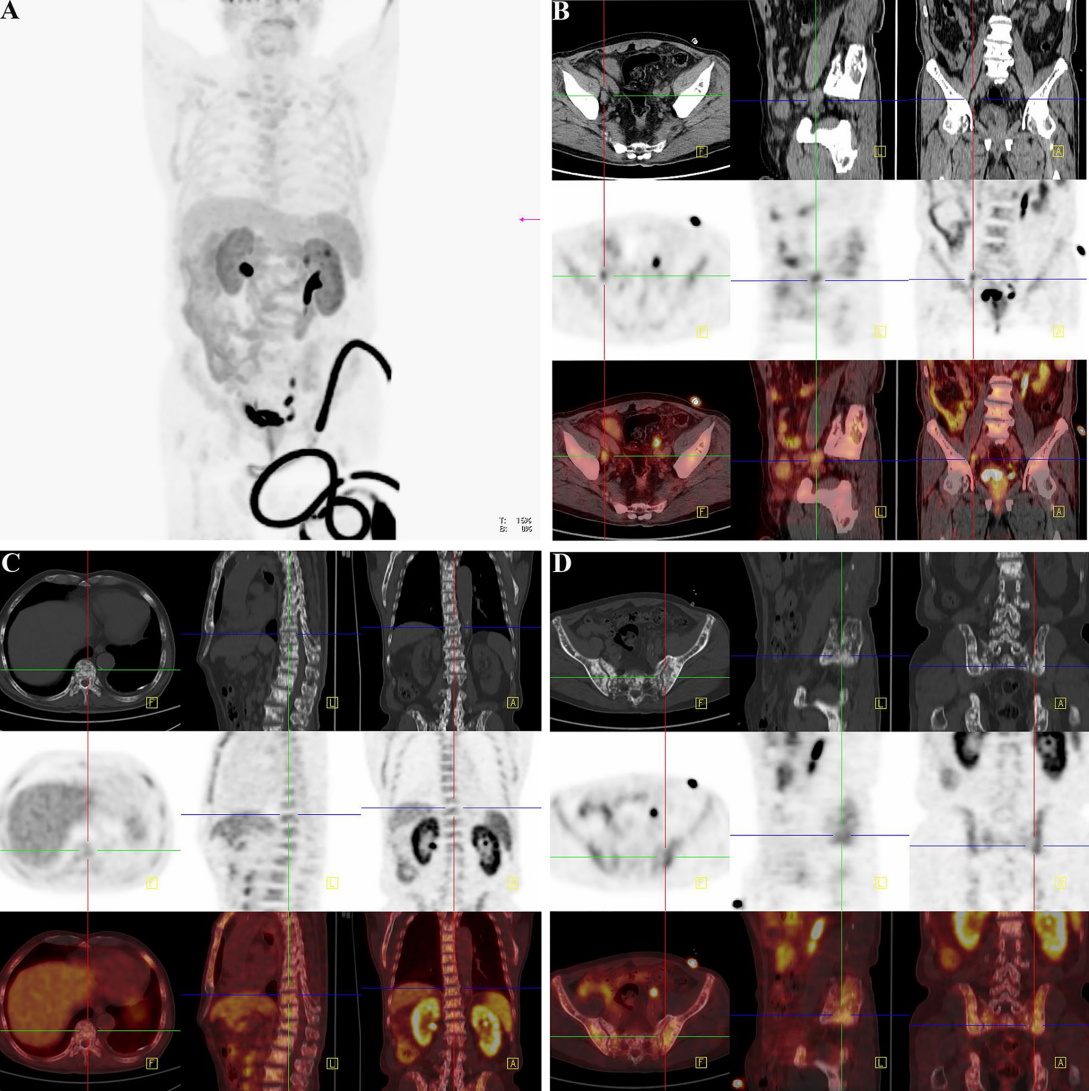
Another clinical replicate case from PET-CT in the case of 76-year-old man. (A) Gross view of coronary position detected by PET-CT. Similarly, the darker the color was, the higher the intake of tracer in the corresponding body part. Since the tracer was excreted through urine, the organs of urinary system were perceptibly high take of tracer. However, the prostate indicated abnormal high intake with dark tracer accumulation, consistent with local lesion of PCa. At the lower right corner of the picture, the spinning black “wire” should be the indwelling urine catheter and the urine with tracer inside. The bladder of the patient was not full, for the existence of urine catheter led the urine into the urine collection bag instantly. (B) The direction cross focused on the enlarged lymph node on the right side of the external iliac vessel. From the view of regular CT, the first row exhibited enlarged lymph node in right side of the external iliac vessel with the same density of soft tissue, and it displayed its neighboring tissues and bone structure. The specific nature of enlarged lymph node was unclear. The high-intake lesion on the right side of the external iliac vessel was also detected by the probe of ^18^F-Alfatide II in the second row from three directions, which suggested convincing evidence of lymphatic metastasis of PCa. Meanwhile, there were other two high intake points in the same slice of axial direction. As the association of the first and second rows, the third row demonstrated the highlighting point on the right side of the external iliac vessel was enlarged lymph node of metastasis while the other two points were a primary tumor lesion in the prostate and a section of urine catheter *in vitro* according to their position. Therefore, except for the tracing of bone metastatic lesion, the utilization of detecting lymph node of metastasis by the probe of ^18^F-Alfatide II should also be available. (C) The direction cross focused on the metastatic lesion in the lumbar 1 vertebra. The first, second, and third rows corresponded to the view of regular CT, PET-CT (^18^F-Alfatide II), and mergence in three directions in the lesion of the lumbar 1 vertebra. The first row presented the high density of bone cortex in the lumbar 1 vertebra, which also tallied with the feature of bone metastasis in PCa. The second row showed the abnormal high intake lesion of ^18^F-Alfatide II in the lumbar 1 vertebra, hinting the malignant tendency of this lesion. As the mergence of the first and second rows, the third row displayed the abnormal lesion in the lumbar 1 vertebra through high brightness signal. (D) The direction cross focused on the metastatic lesion behind the left ilium. The first, second, and third rows separately revealed the observed lesion behind the left ilium in the view of regular CT, PET-CT (^18^F-Alfatide II), and combination of regular CT and PET-CT in three directions. In the first row, we could find the unusual bone hyperplasia behind the left ilium. Then, the high intake of tracer in the views of PET-CT (^18^F-Alfatide II) revealed malignant metastatic lesion. The third row provided the combination of the first and second rows that we could discover corresponding region of another bone metastatic lesion.
